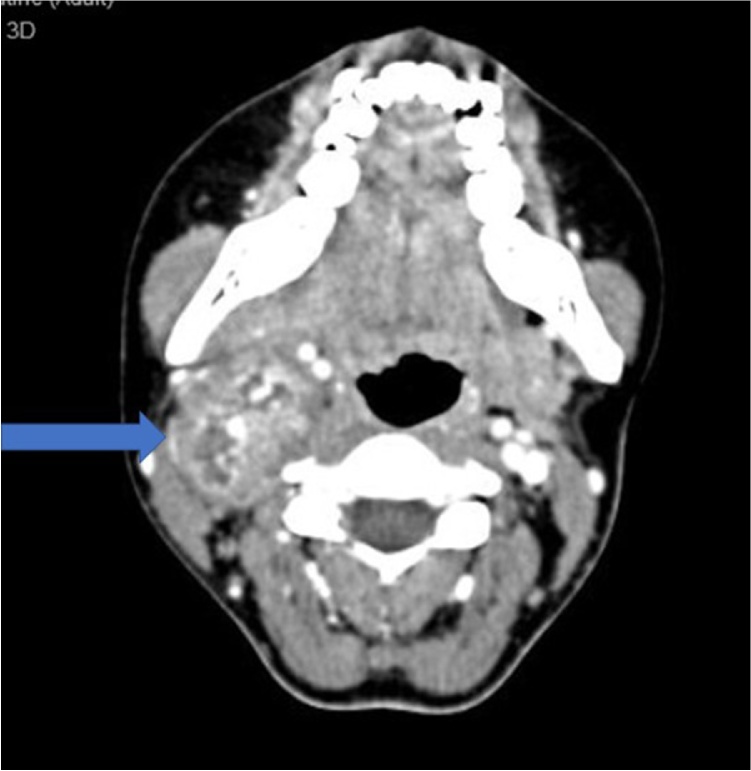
CT scan of the neck showed a heterogenous, well encapsulated tumor which located between the carotid artery and the vagal nerve.
